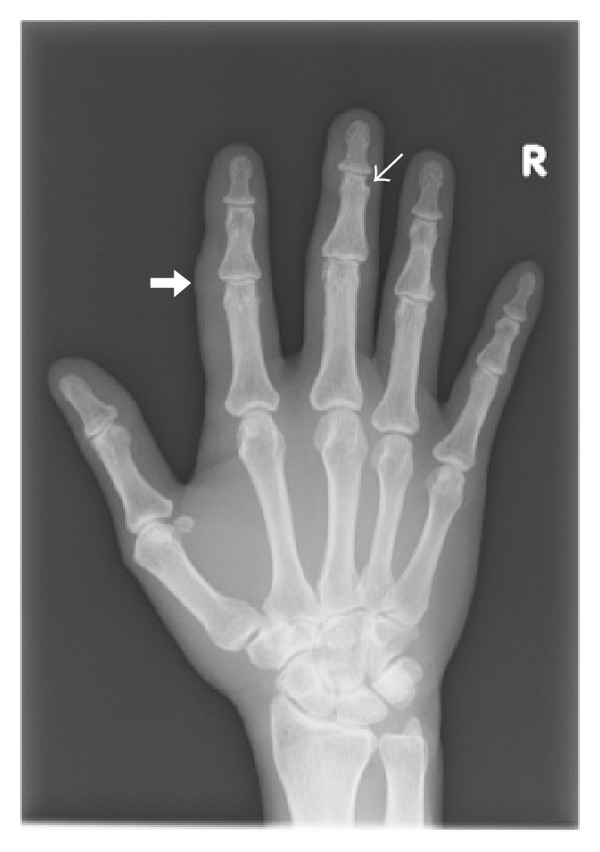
Frontal radiograph of the right hand shows focal periarticular soft tissue swelling at the proximal interphalangeal joint of the 2nd digit (thick arrow), suspicious for dactylitis. A small juxta-articular erosion is also noted at the middle phalanx of the middle finger (white arrow), which may represent the arthropathic changes related to HS.
